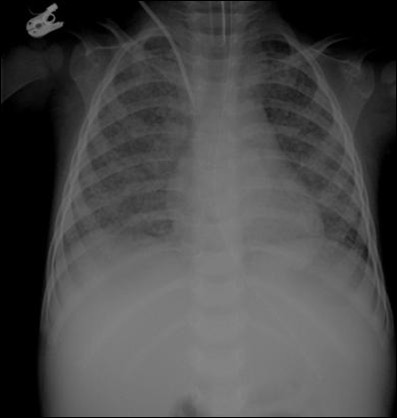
Chest X-Ray. Diffuse, bilateral, small lung nodules suggestive of acute miliary pulmonary tuberculosis.
